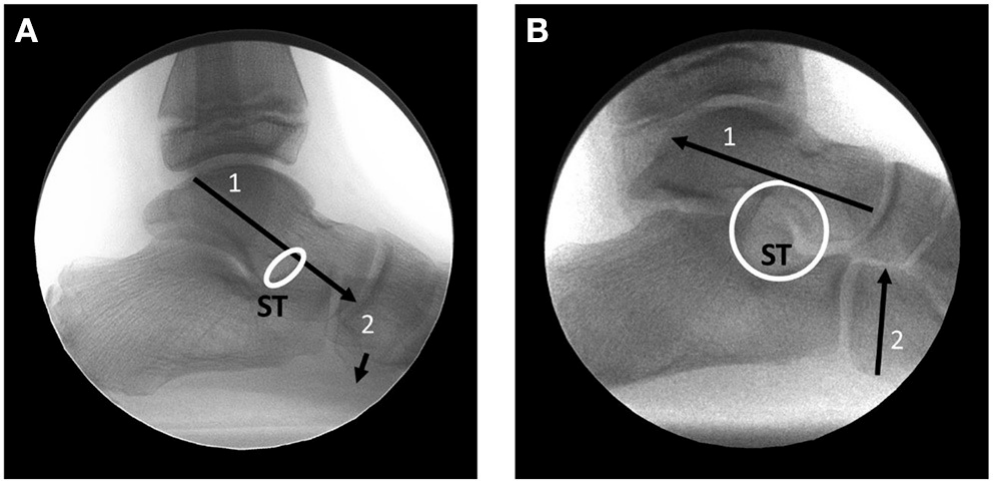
Lateral weight bearing fluoroscopic radiographs. (A) Relaxed stance position. The talus has partially dislocated on the calcaneus (1). The sinus tarsi (ST) is obliterated and the navicular has dropped (2). (B) The same foot with the articular facets of the talus repositioned on the calcaneus (1). Sinus tarsi (ST) is re-opened and the navicular is elevated (2).
